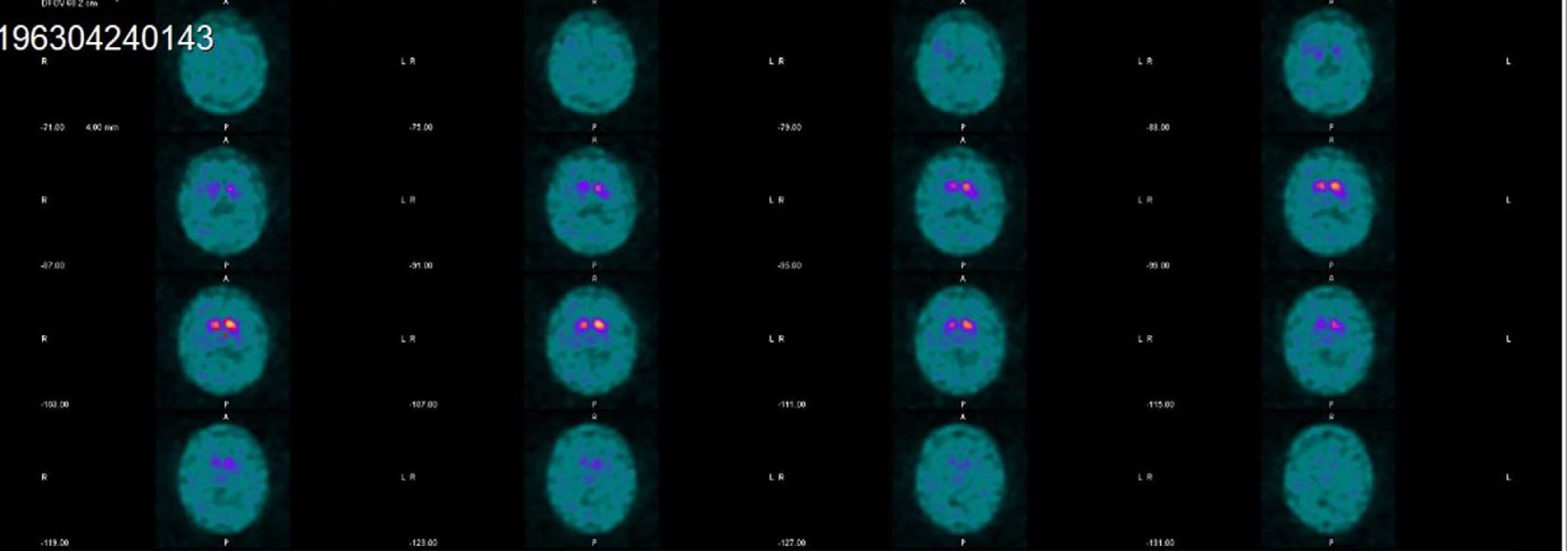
(DAT [dopamine transporter]) SPECT (single‐photon emission computed tomography) scan showing bilateral reduction in dopamine transporter isotope in the putamen more marked on the left and compatible with nigrostriatal dopamine denervation.
